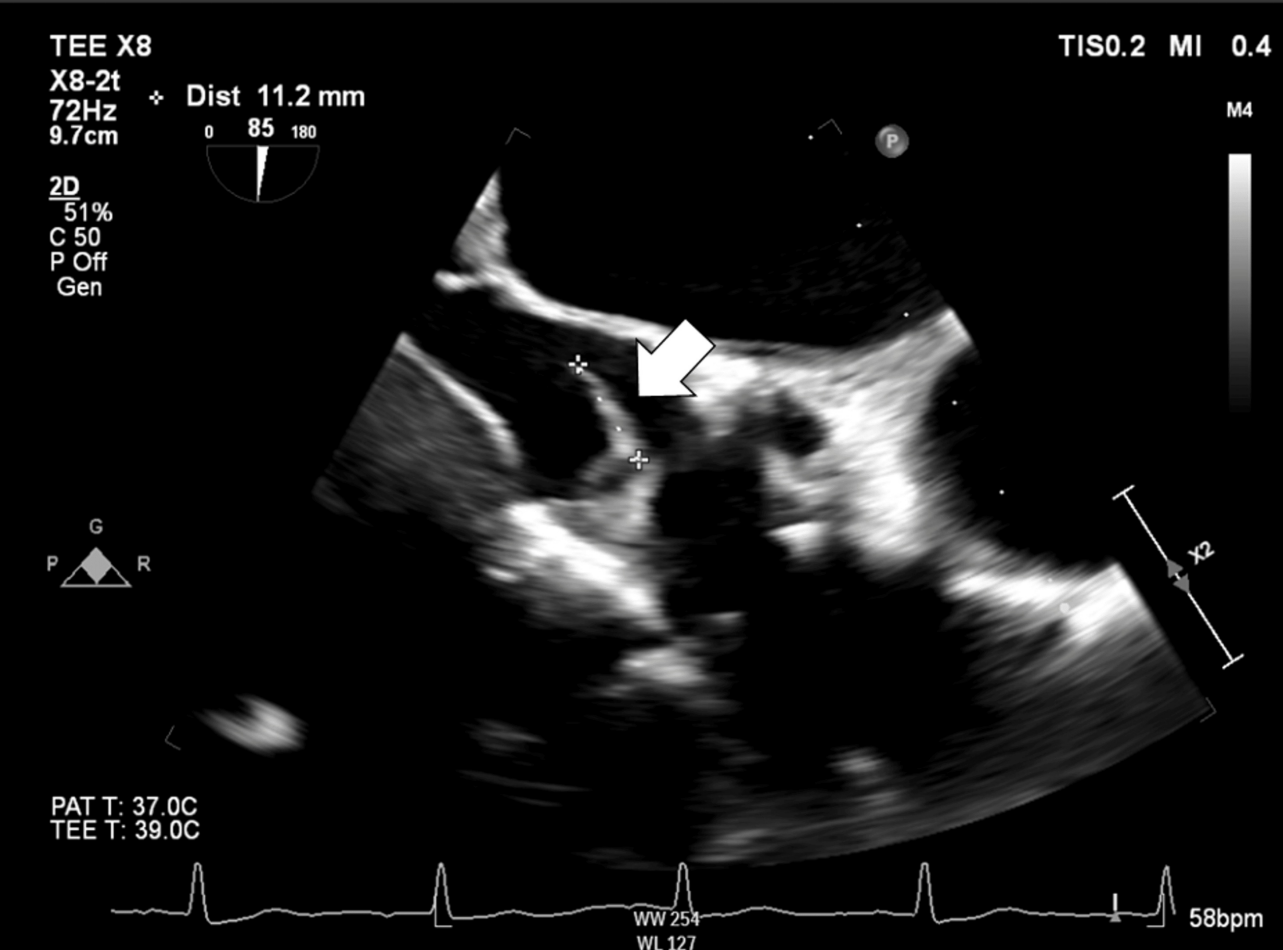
Transesophageal Echocardiography Findings A mobile, linear structure measuring 11.2 mm is observed on the transcatheter aortic valve prosthesis (white arrow), consistent with vegetation due to infective endocarditis.
